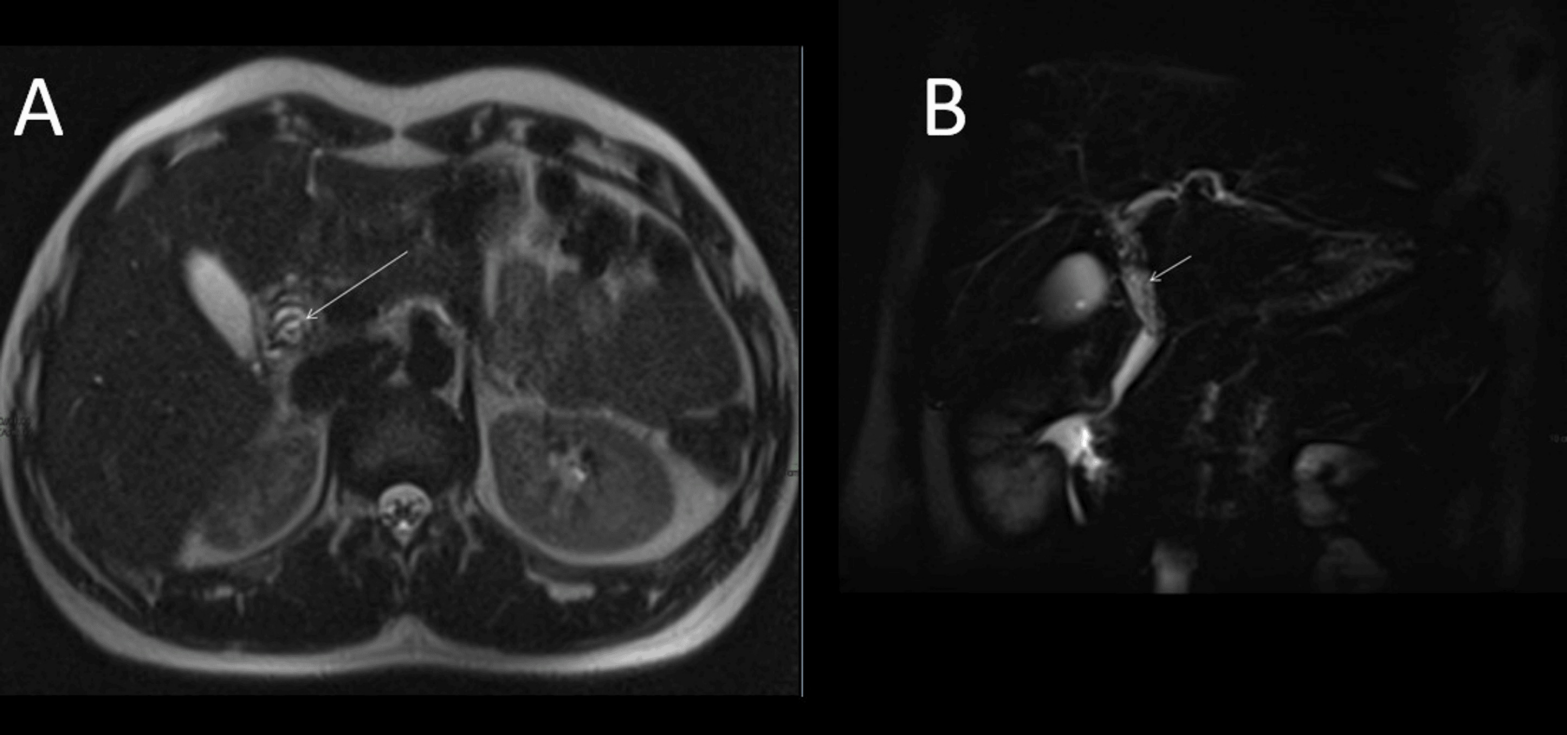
Magnetic resonance cholangiopancreatography (MRCP) images (A) Axial T2-weighted images reveal linear low-signal intensity within the common bile duct (CBD) (arrow). (B) Coronal T2 fat-saturated images also demonstrate linear low-signal intensity within the CBD (arrow).
